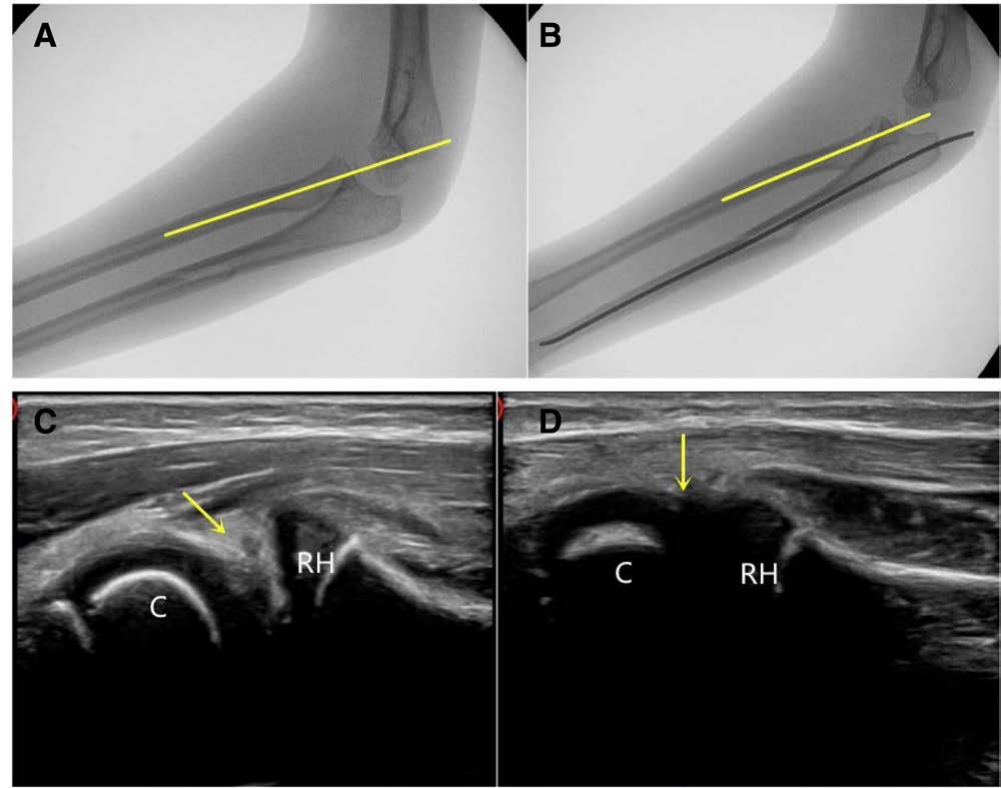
(A) A 5-yr-old girl, the fracture of the ulna was displaced on the X-ray lateral view, and the axis of the radial neck passed through the upper 1/3 of the capitellum (arrow). (B) The axis of the radial neck passed through the middle 1/3 of the capitellum after ultrasound monitoring closed reduction. (C) Ultrasound showed obvious displacement of the radial head with soft tissue incarceration (arrow) before reduction. (D) Ultrasound showed normal “double-hump sign” and “congruency sign” after closed reduction (arrow). C = capitellum, RH = radial head.
